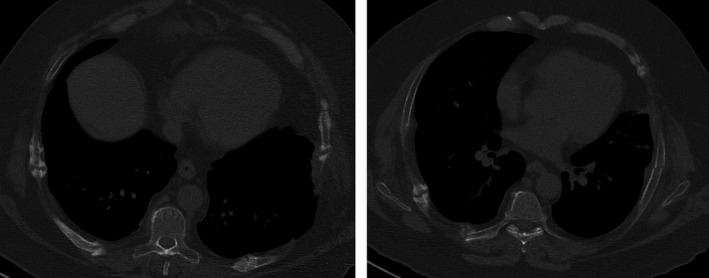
High‐resolution chest CT with evident fractures of costal arches
